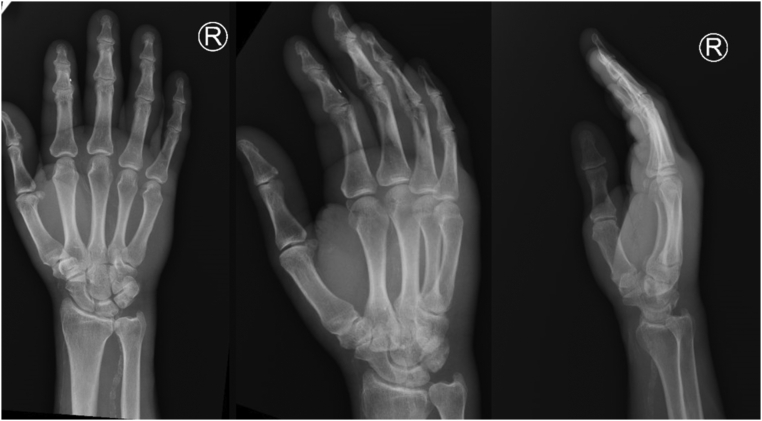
Right hand radiographs showing soft tissue swelling extending to the fourth digit and normal bone anatomy (from left to right: AP, Oblique, Lateral views).
